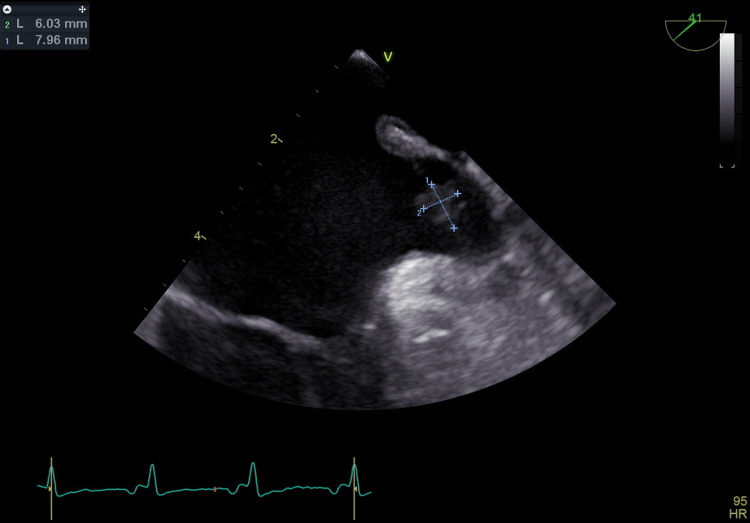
Transesophageal echocardiogram (case 2) showing left atrial appendage vegetation
